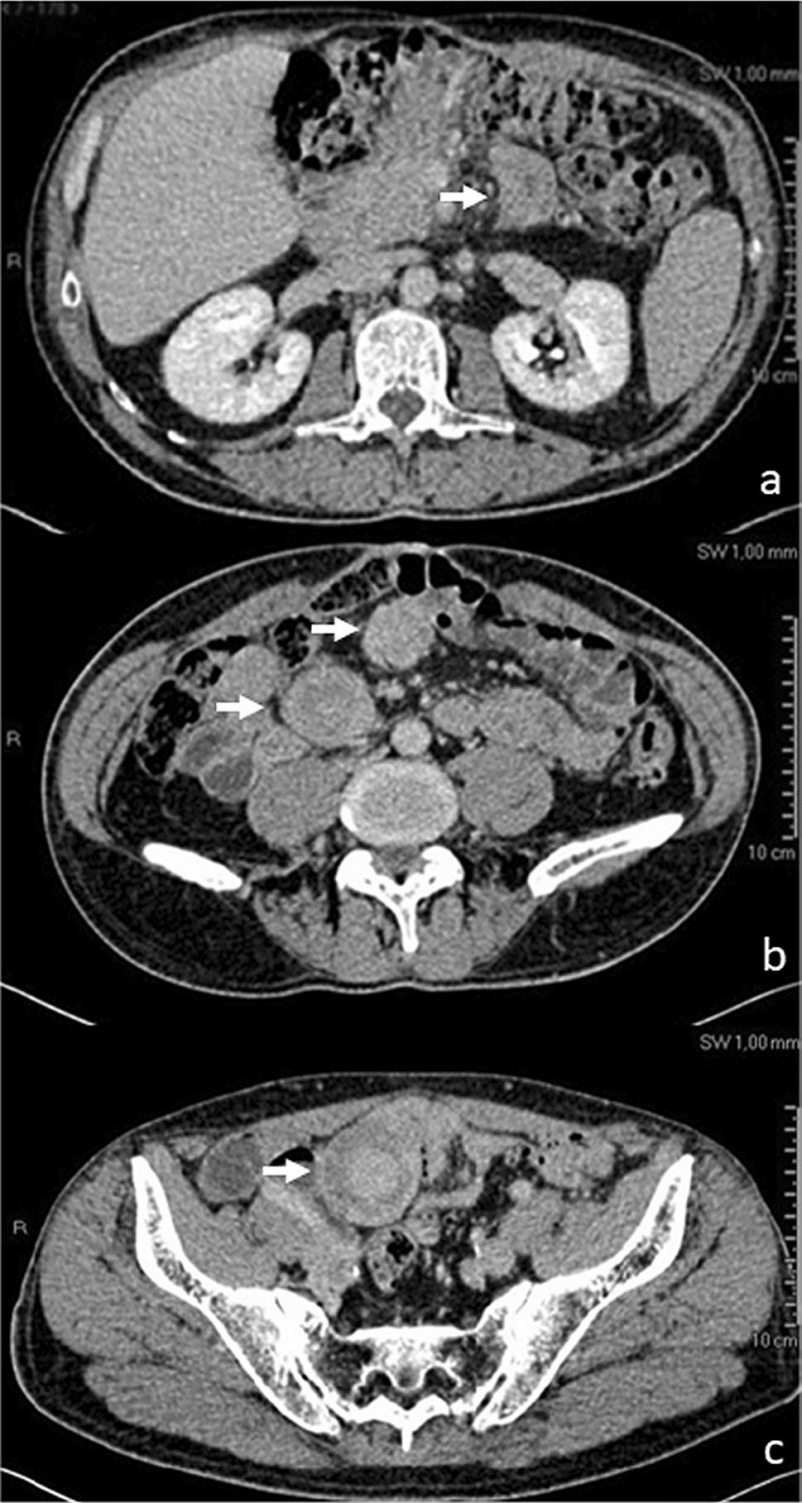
Contrast CT scan eighteen months after laparotomy for walled off pancreatic necrosis, showing four discrete intraabdominal masses (arrows), ranging from 1.6 to 4.8 cm diameter, isoattenuating to hyperattenuating to muscle
